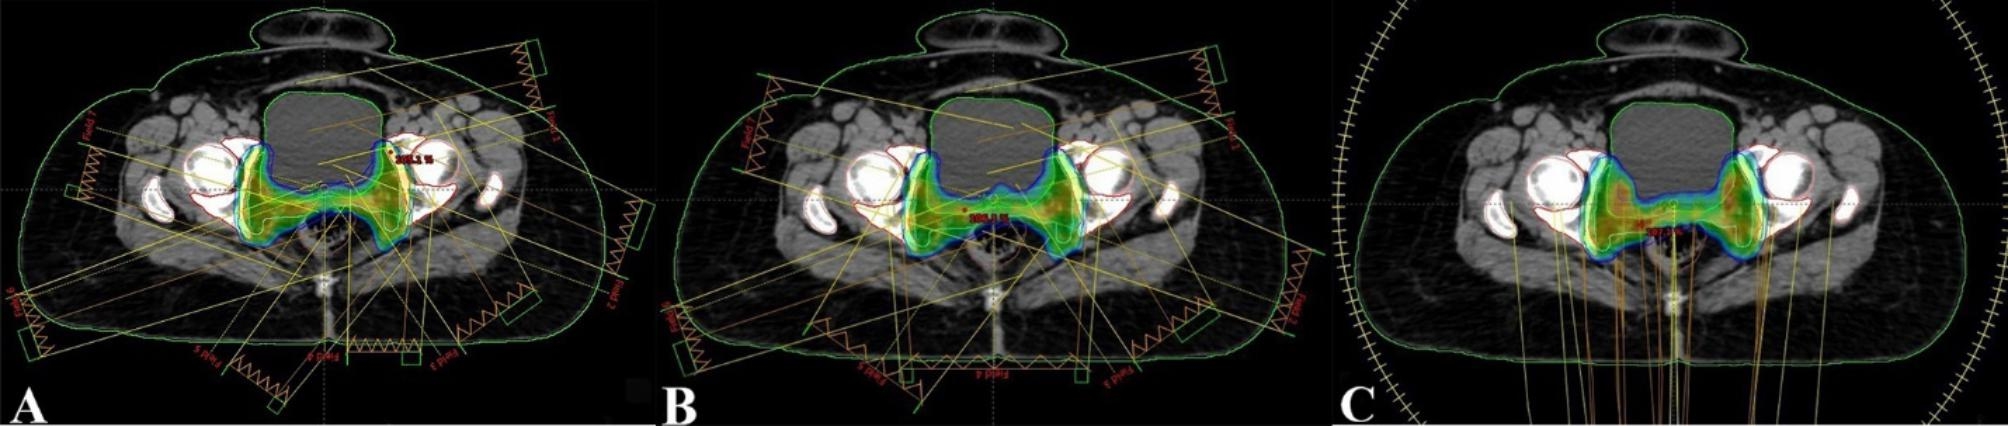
Axial computed tomography scan slice representations of a patient planned with C-IMRT (A), A-IMRT (B) and VMAT (C). Axial view of the 95% isodose of the prescribed dose in dose color wash, PTV (dark blue), CTV (cyan), bladder (green), rectum (brown) and femoral heads (pink) were shown
